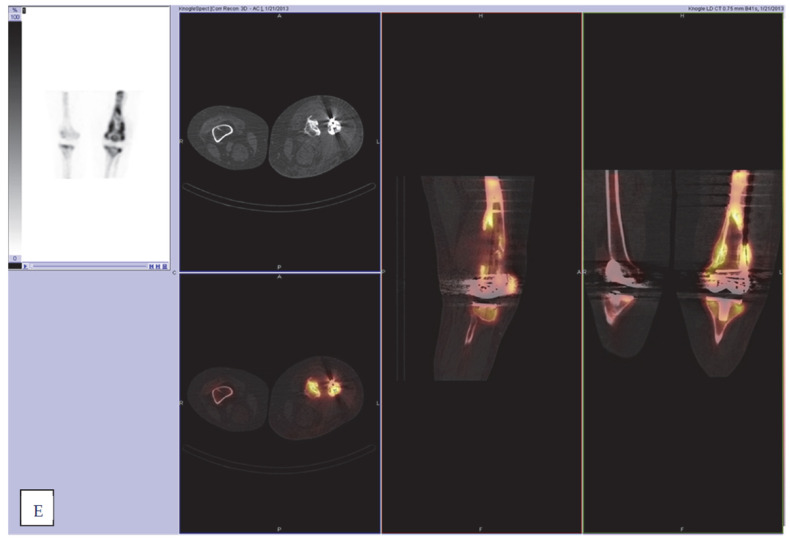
Three hybrid imaging modalities for the same patient with an infected knee prosthesis. (A) FDG PET/CT (showing high FDG uptake located in distal femoral bone marrow and around cortical bone defects, also around the screws in lateral femoral condyle (arrows)). (B,C) Dual-isotope combined In-labeled leucocyte SPECT/CT (B) and bone marrow SPECT/CT (C) (showing mis-matched focal leucocyte uptake (arrow) in distal femoral bone marrow and around cortical bone defects, also around the screws in lateral femoral condyle). (D,E) Bone scan; static uptake (D), SPECT/CT (E) (showing generally high uptake in bone tissue around the prosthesis and metal fixation both in distal femur and proximal tibia).
